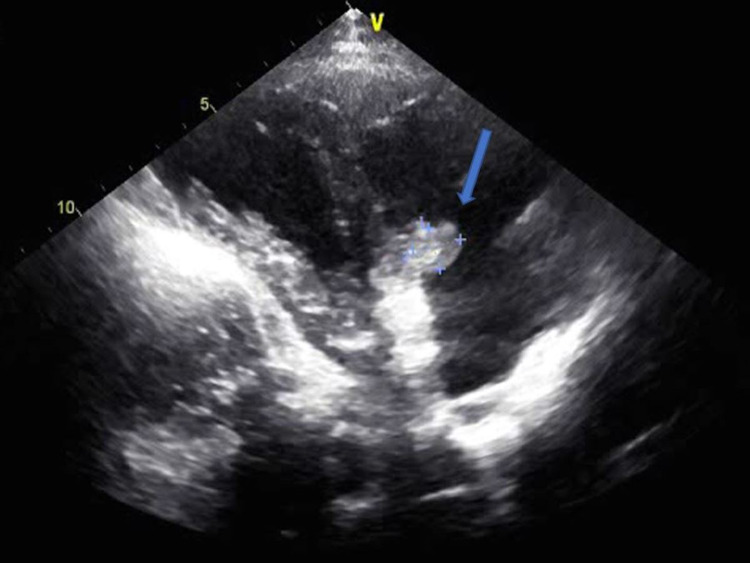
Transthoracic echocardiogram short-axis view Blue arrow showing tricuspid valve vegetation
